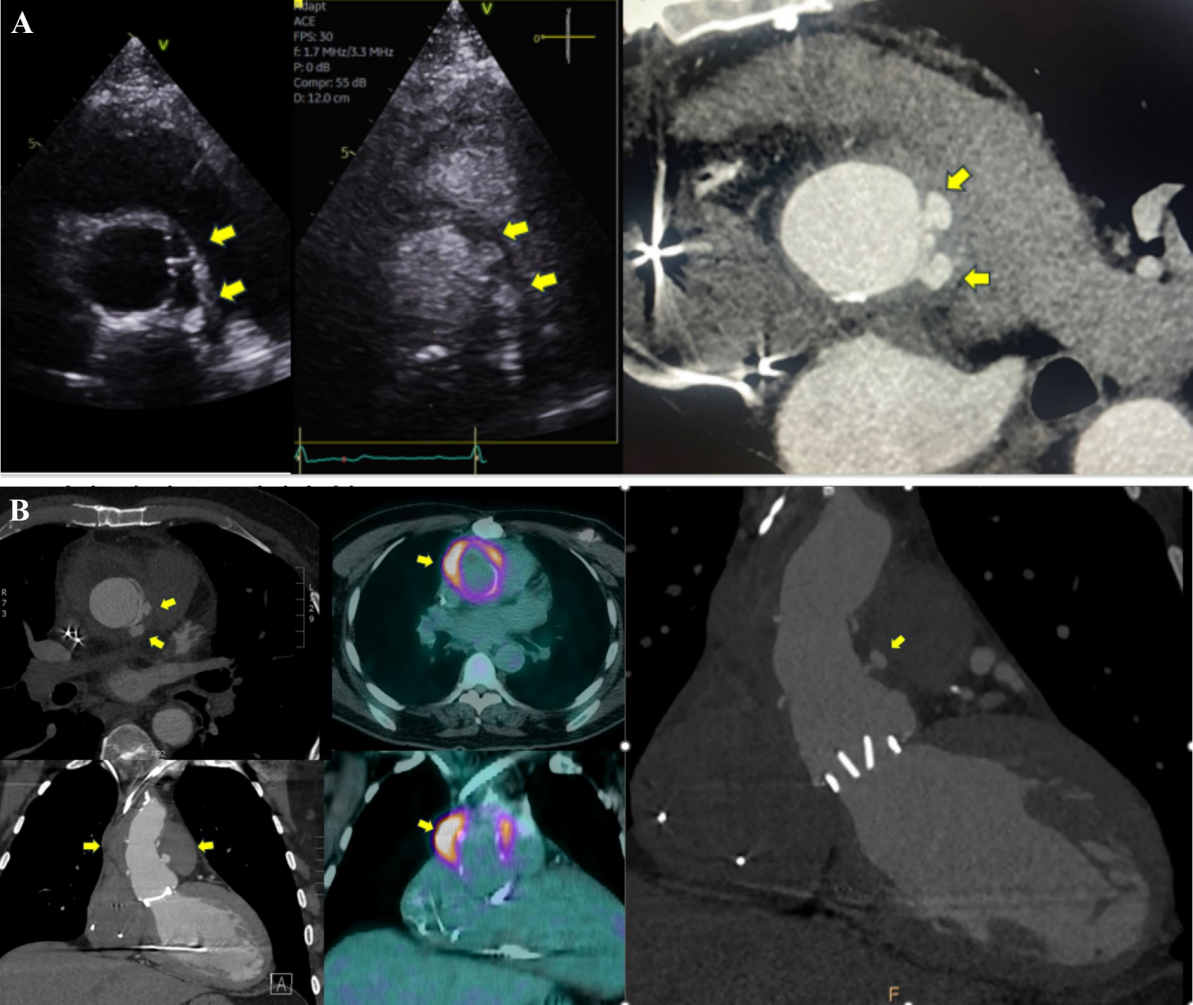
63-year-old gentleman with prior mechanical aortic valve replacement and a supra-coronary ascending aortic graft five years prior, presenting with *Methicillin-Sensitive Staphylococcus Aureus* (MSSA) bacteremia and suspected mechanical aortic valve endocarditis. Transthoracic echocardiogram revealed no vegetation but did show an echolucent space around the ascending aorta graft. Transesophageal echocardiogram could not be performed due to grade D esophagitis and ulcers. The patient was also found to have multifocal cerebritis and small cerebral abscesses on CT brain imaging. A subsequent CT cardiac angiogram revealed a perigraft collection around the ascending aorta graft with two small pseudoaneurysms at the site of the anastomosis between the native aortic root and the supra-coronary ascending aorta graft junction, correlating with the echolucent space seen on echocardiography. FDG-PET imaging confirmed infection with FDG uptake around the graft. Figure 5A shows a side-by-side comparison of the perigraft echolucent space on standard echocardiography, echocardiography with Definity contrast, and CT coronary angiogram (A, B, and C, respectively). Figure 5B reveals the ascending aorta pseudoaneurysm and perigraft collection in both short-axis and long-axis images, as well as FDG uptake on PET imaging in this region. The patient was referred for surgery
